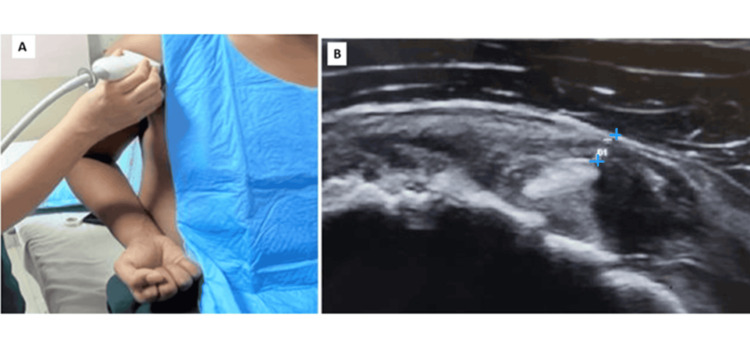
Measurement of the rotator interval thickness by ultrasonography A) Position of the probe; B) Ultrasound image
